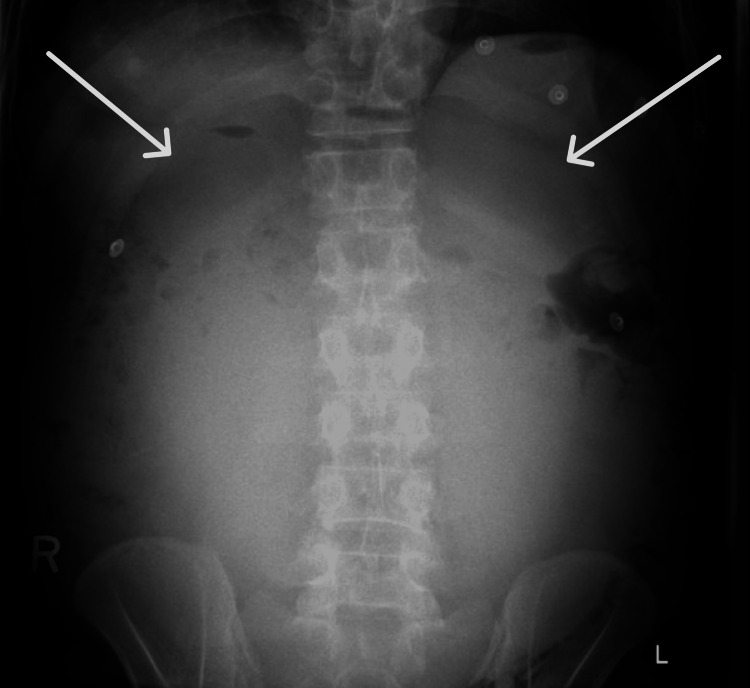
Anteroposterior (AP) abdominal X-ray, erect position White arrows indicate areas of diffuse abdominal opacity, suggestive of a mass
